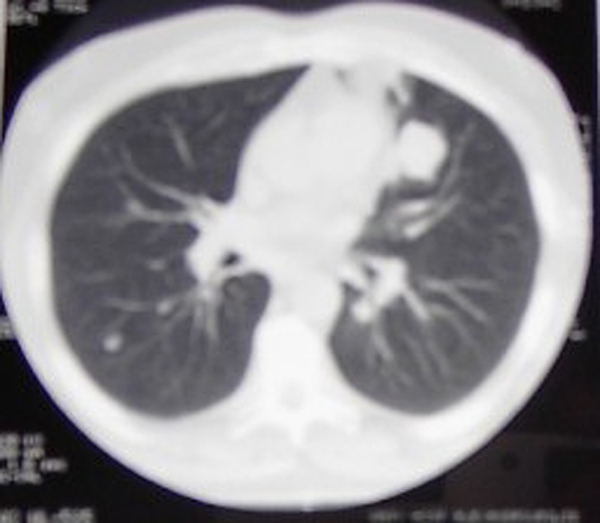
CT scan of the chest shows a tumour mass in the left lung.
